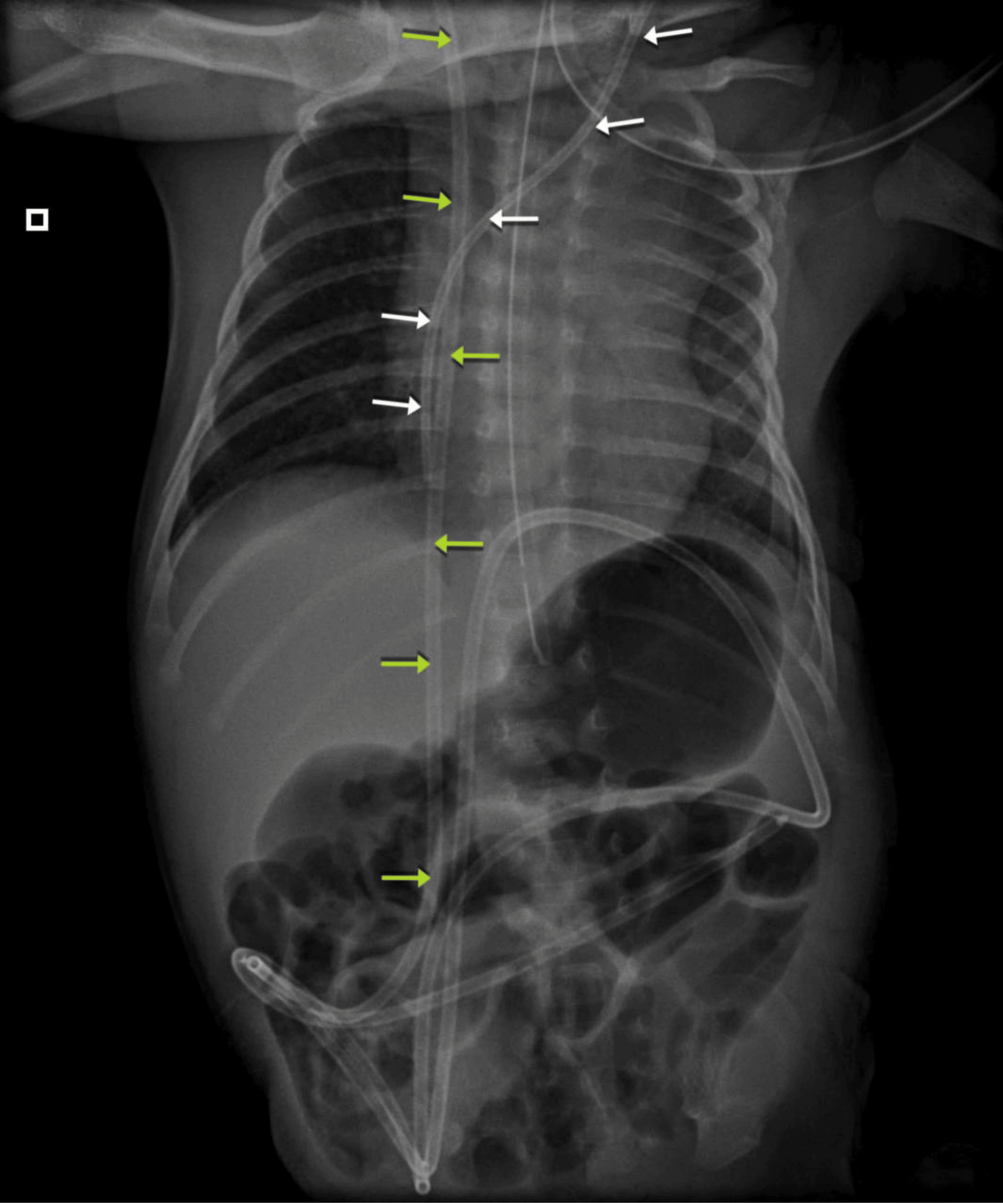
Anteroposterior chest and abdominal X-ray White arrows indicate the path of the central venous catheter. The thoracic and abdominal course of the ventriculoperitoneal shunt is also visible, marked by the green arrows.
